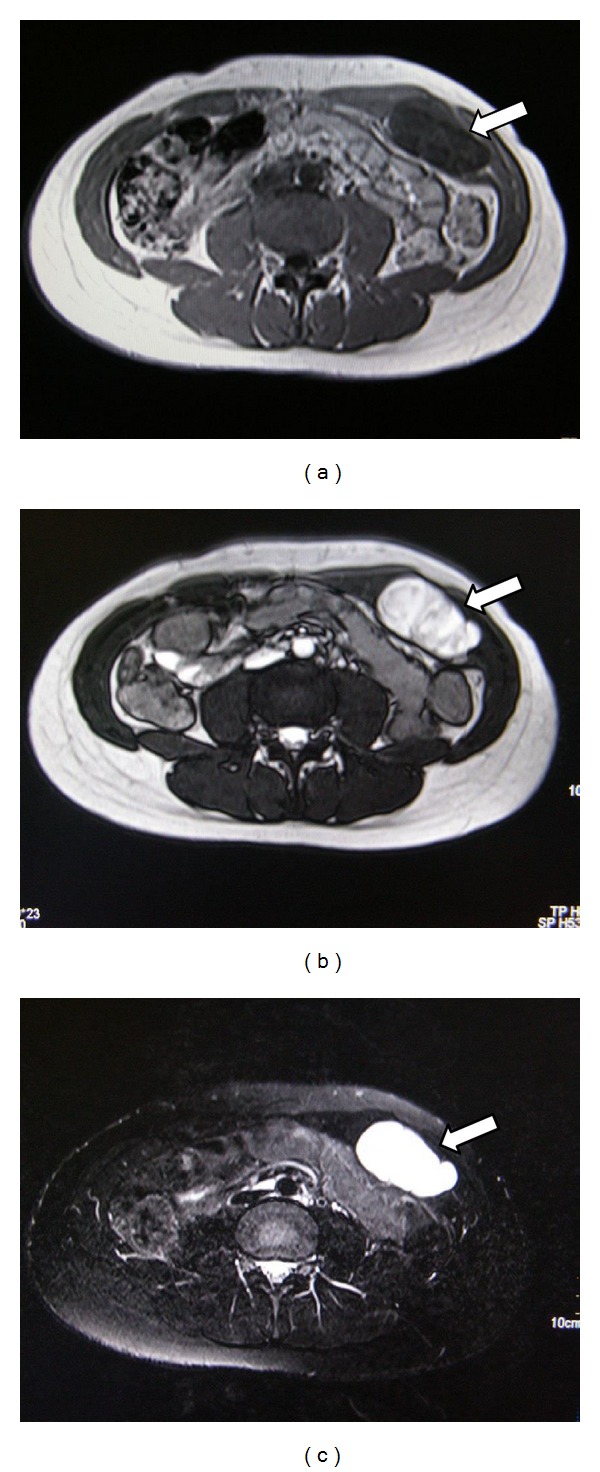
(a) T1W MRI shows encapsulated superficial mass with marked heterogeneity and patchy hypointense foci (arrow) suggesting cystic degeneration. (b) T2W MRI clearly delineates the capsule of the lesion and internal morphology (arrow). (c) The lesion is markedly hyperintense on fat-suppressed MRI suggesting degeneration (arrow).
